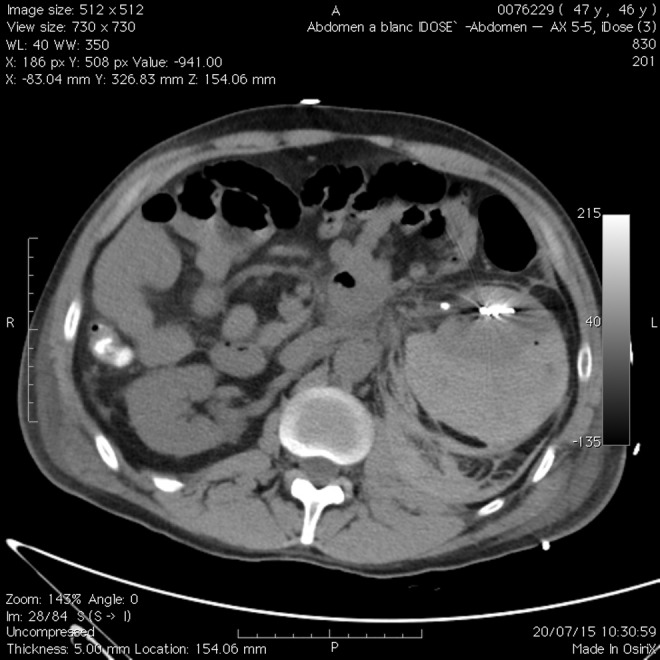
Axial CT image of a resorbing massive subcapsular and retroperitoneal renal hematoma in the left hemiabdomen, caused by a third consecutive SWL treatment of a calculus in the left UPJ, after coiling of the bleeding site.
